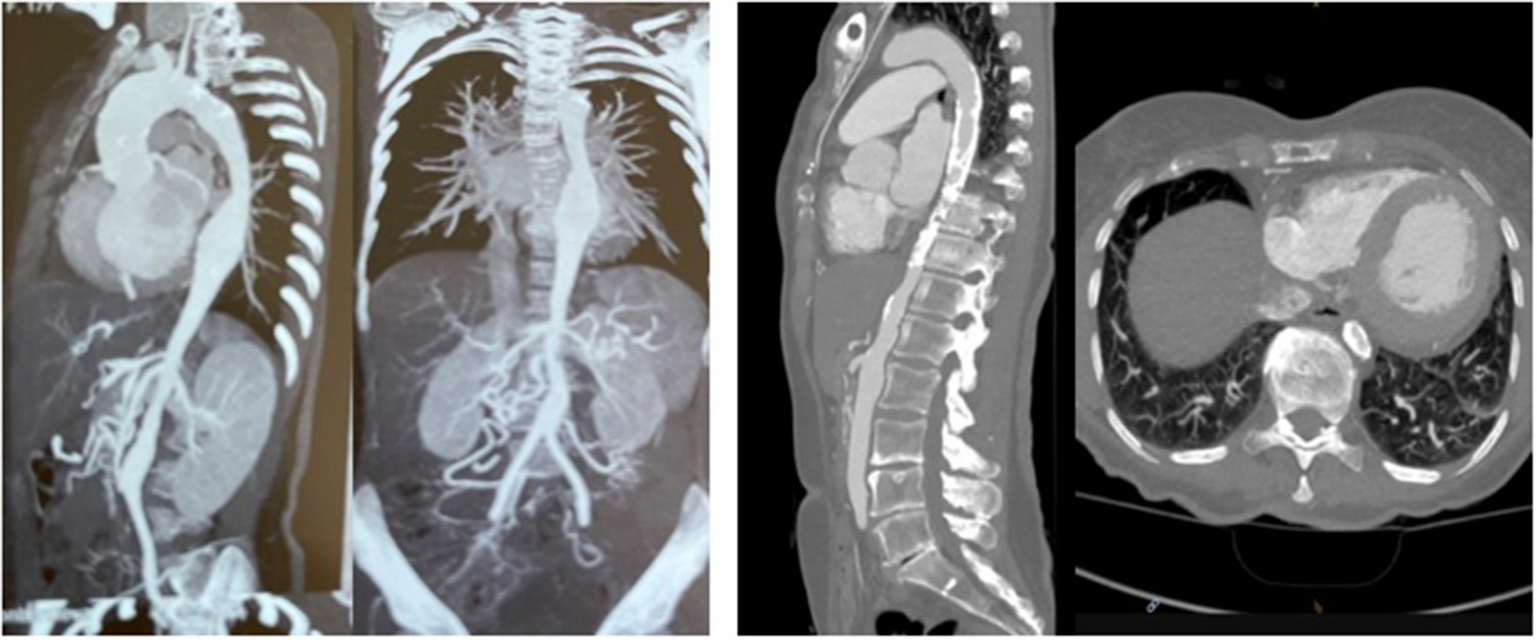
Left (2 pictures): CT-angiogram of a young patient with Takayasu aortitis with multiple stenoses in the thoracic and abdominal aorta and occlusion of the iliac arteries scheduled for an descending to bi-iliac extra-anatomic bypass. Right (2 pictures): CT-angiogram of a 45 yr-old female with severe calcific narrowing of the descending aorta as a result of a Takayasu more than 25 years before. Endovascular procedure was thought to be not ideal in this case.
